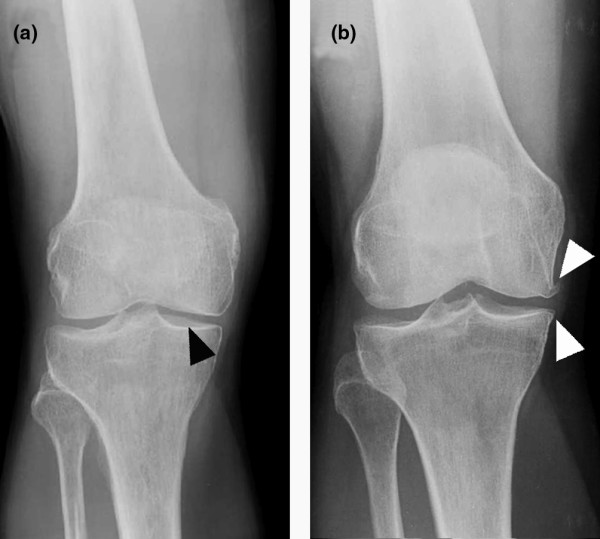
Radiographs of patients with focal degenerative cartilage defects prior to treatment with autologous chondrocyte grafts (BioSeed^®^-C). (a) This patient showed a Kellgren-Lawrence score of 2, with narrowing of the joint space (black arrowhead). (b) This patient showed a Kellgren-Lawrence score of 3, with narrowing of the joint space and osteophytes (white arrowheads).
